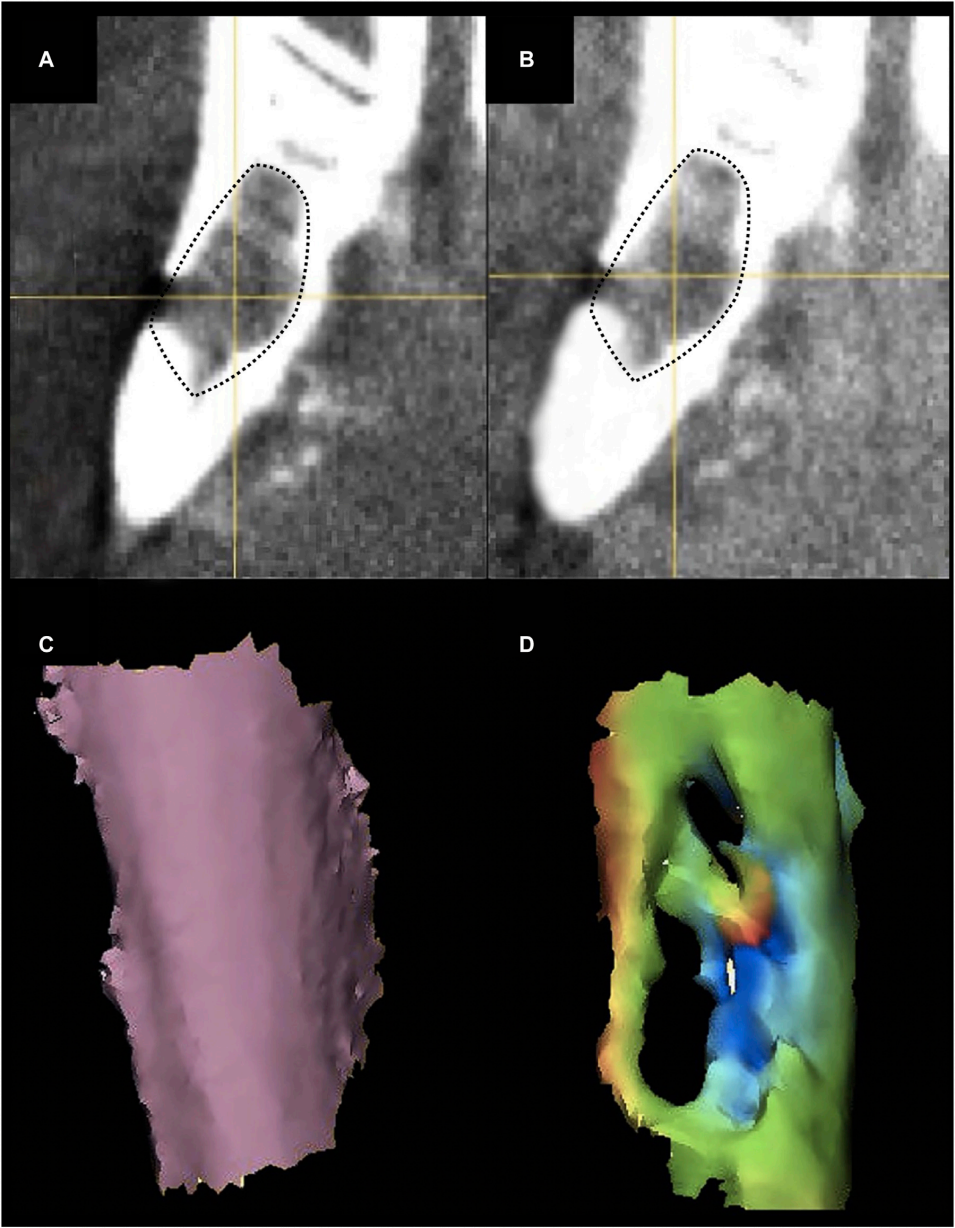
(A, B) Pre- (A) and post-contrast (B) agent injection CT scan of the mandibular defect. Contrast enhancement can be appreciated in the defect area (black dotted-line). (C, D) Example of preoperative cortical shape (C) and 30-day postsurgical cortical shape (D) of the defect area. A color-scale map quantifies the morphological similarity between postsurgical and presurgical shapes (green areas are similar to the original shape, orange-to-red areas are excessively protruding with respect to the original shape, blue areas are depressed with respect to the preoperative shape).
